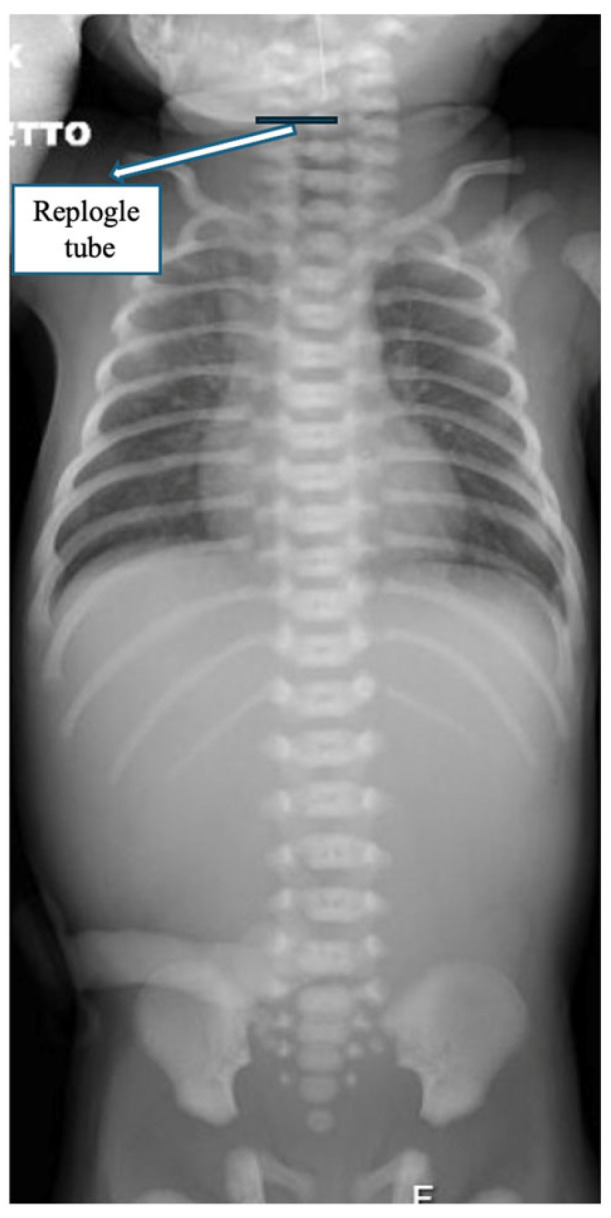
The esophageal gap and the X-ray showing the replogle tube in the neck and the absence of intra-abdominal gas.
